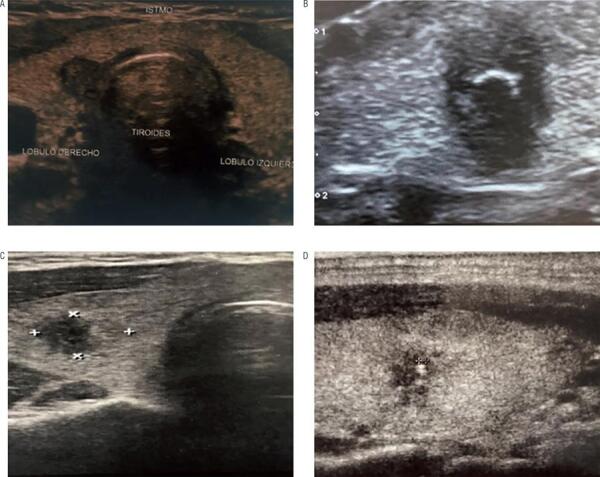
Ultrasonographic images of selected patients with PTC undergoing active surveillance.
